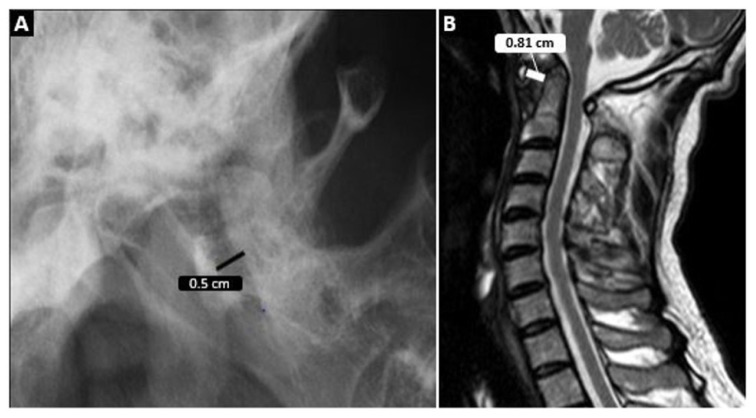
Atlantoaxial distance measurement on conventional radiography is seen in A and MRI in B.
